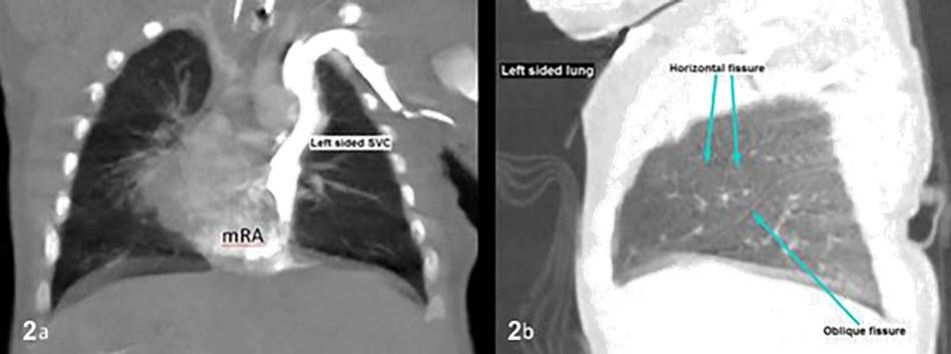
CT thorax confirmed atrial situs inversus whereby there was single left-sided SVC draining into the left-sided mRA as shown on coronal plane (a) and trilobed left lung as shown on sagittal plane in lung window (b). The pulmonary veins drain into the right-sided mLA (image not shown). Inferior vena cava was poorly demonstrable. SVC, superior vena cava; mRA, morphologic right atrium; mLA, morphologic left atrium.
